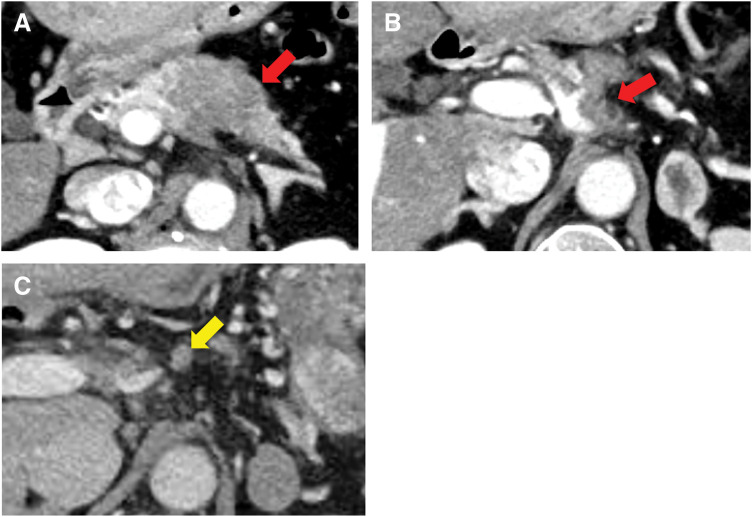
(A) Contrast-enhanced CT showing a 38-mm hypovascular tumor (red arrow) in the body of the pancreas. (B) The tumor had invaded the celiac (red arrow), splenic, and common hepatic arteries. (C) Before treatment, the regional lymph nodes appeared slightly prominent (yellow arrow).
